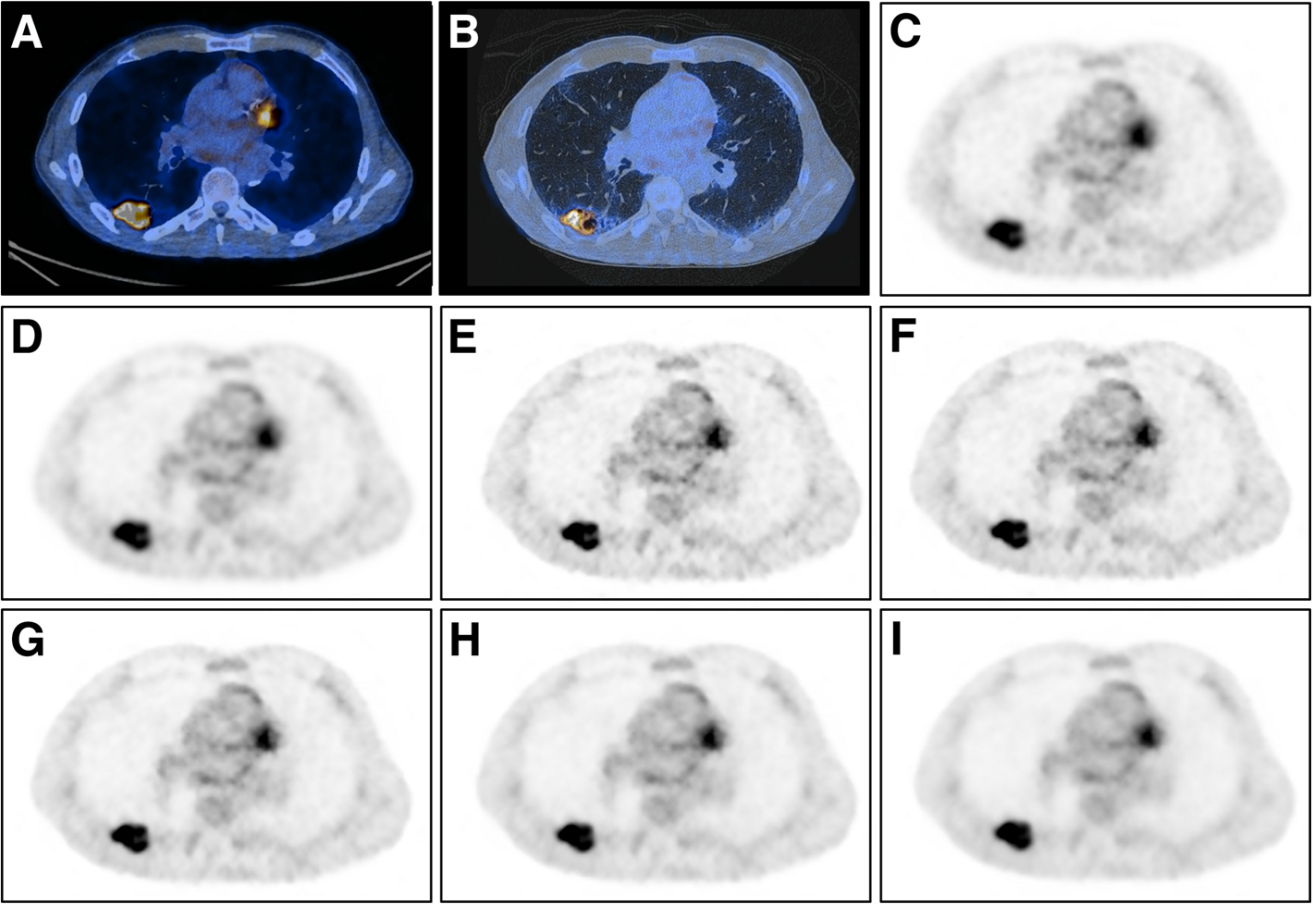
Representative images of a 66-year-old man with a body mass index of 20.7 kg/m^2^ and 59 kg body weight who underwent 18F-FDG PET/CT for staging of lung cancer. The patient was injected with 117.9 MBq of 18F-FDG (i.e., 2.0 MBq/kg body weight), according to the BMI-adapted dosage protocol developed for digital PET [14]. Co-registered PET/CT images (a, b) show a highly 18F-FDG-avid tumor in the right lower lobe, which was confirmed as adenocarcinoma after wedge resection. Axial PET images are given in c–i, showing OSEM_TOF_ (c), OSEM_PSF_ (d), BSREM_350_ (e), BSREM_450_ (f), BSREM_600_ (g), BSREM_800_ (h), and BSREM_1200_ (i)
